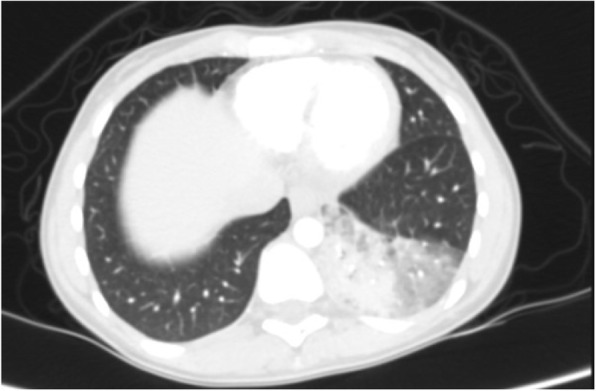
Streptococcus pneumonia: thin section CT shows segmental consolidation and GGO in left lower lobe
